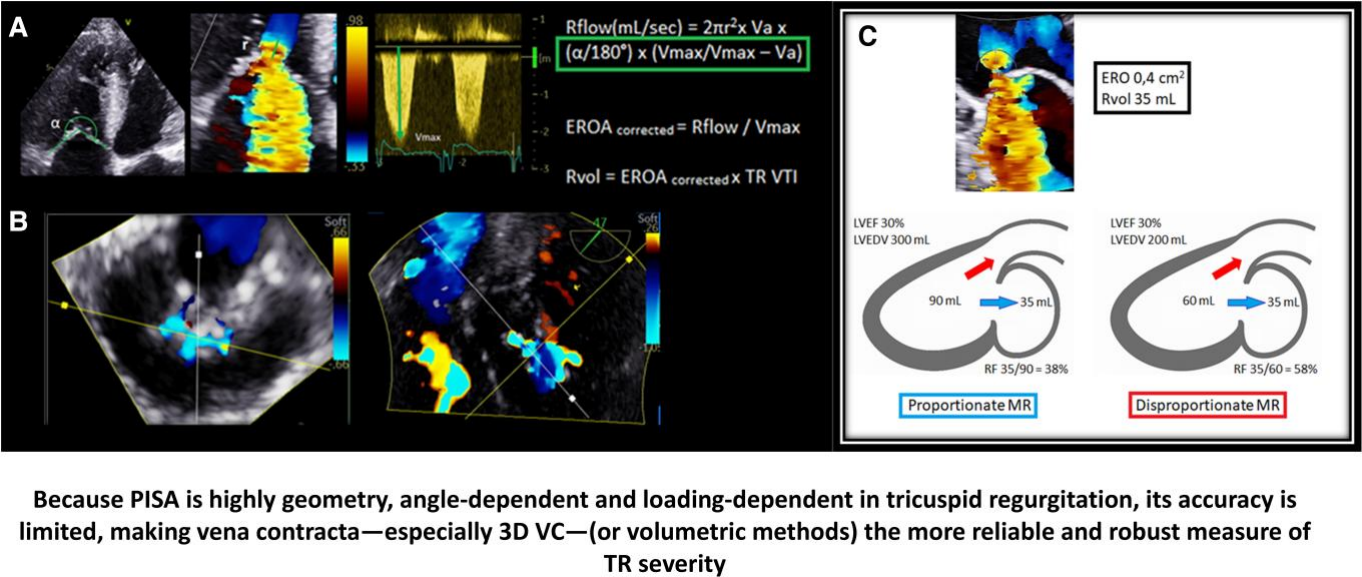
Advanced quantification of functional regurgitation: corrected PISA and 3D orifice morphology (Panel *A*): corrected PISA method for tricuspid regurgitation, using angular (α/180°) and velocity (*V*_max_/*V*_max_-*V_a_*) adjustments to account for non-hemispheric convergence and aliasing underestimation. Key measurements include flow radius (*r*), and CW Doppler *V*_max_, used to derive corrected EROA and Rvol. (Panel *B*): En face 3D colour Doppler views showing a slit-like elliptical mitral orifice (left) and an irregular, multi-lobed tricuspid orifice (right). These geometries challenge the assumptions of circular PISA and highlight the utility of 3D vena contracta area (VCA) in functional regurgitation assessment. (Panel *C*). Proportionality model for functional regurgitation. EROA, effective regurgitant orifice area.
